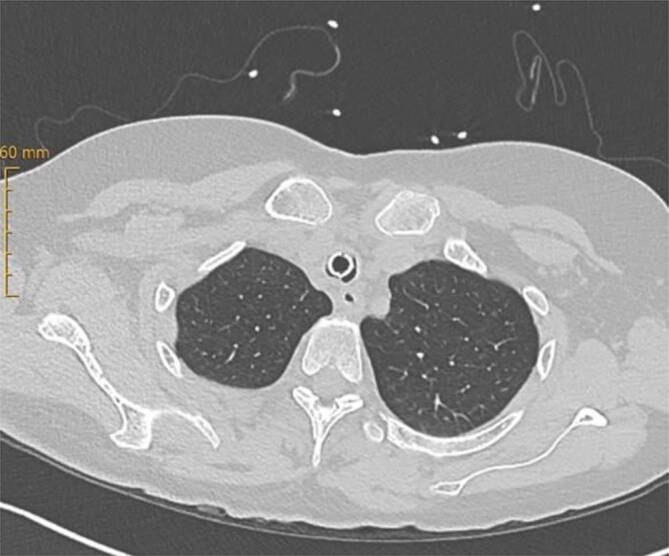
CT-Befund: Fehlen eines Luftemphysems
